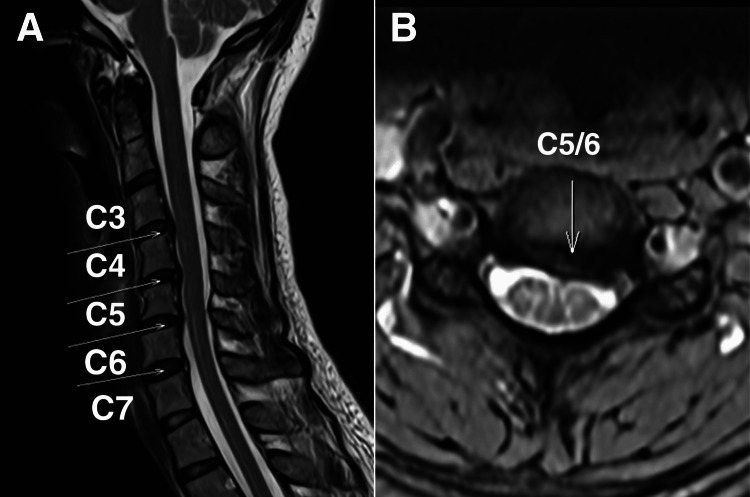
Cervical magnetic resonance imaging, T2-weighted sagittal view A) There is evidence of degenerative cervical myelopathy, including diffuse disc desiccation seen from C2/3 to C6/7 levels with mild loss of disc height at C5/6 level. C4/5 and C5/6 disco-osteophytic complex (white arrows) cause mild spinal canal stenosis and mild spinal cord deformation. B) C5/6 disco-osteophytic complex causes mild spinal canal stenosis and mild spinal cord deformation, more on the left (white arrow).
